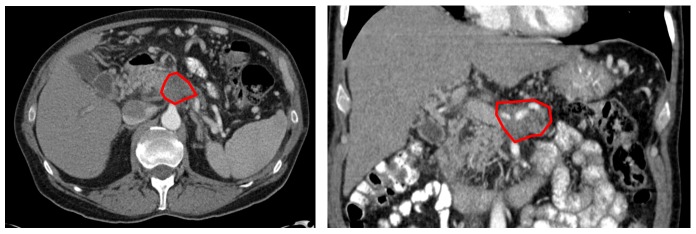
Diagnostic computed tomography with visible pancreatic body lesion.
